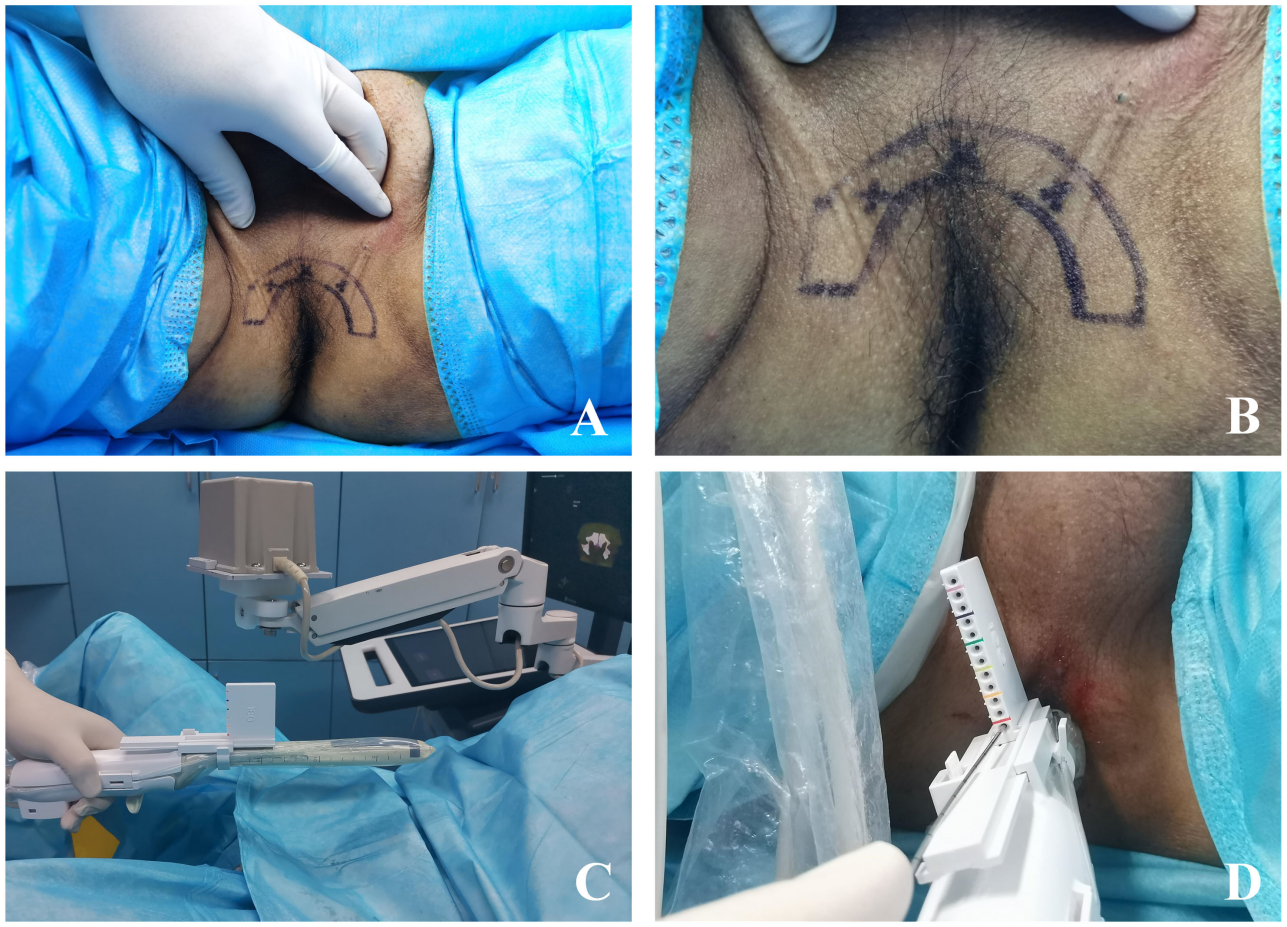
The exterior view of mpMRI-TRUS image fusion targeted TPB using electromagnetic needle tracking under LA. (A) One plane and three-point LA projection mark on the body surface, wherein the fan-shaped semicircle outline is the needle entry area of prostate puncture, and the three forks are the needle entry points of prostate nerve block; (B) Partial enlarged view of the projection mark on the body surface of one plane and three-point LA; (C) The ultrasound probe puncture frame is fixed on the intracavity biplane ultrasound probe, and the electromagnetic navigation tracking system is adjusted to about 10 cm above the surface projection of the prostate; (D) External view of targeted TPB.
